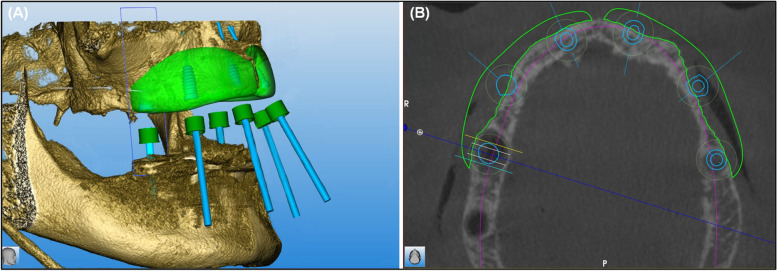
Digital planning based on a 3D X-ray image (DICOM file) for the design of the planned allogeneic bone blocks, including optimised subsequent implant position
